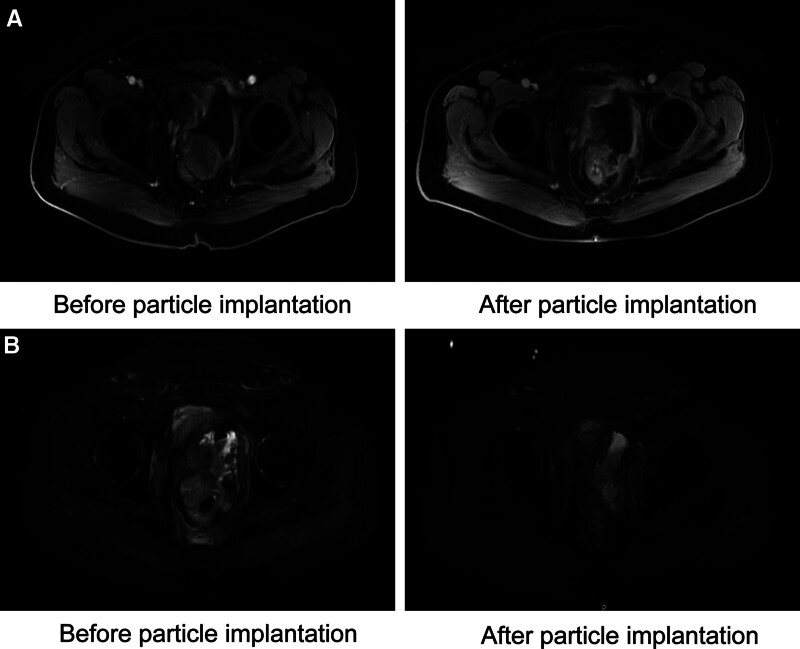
(A) MRI comparison of local recurrent pelvic lesion before I-125 seed implantation and after I-125 seed implantation for 2 months. (B) MRI comparison of second local recurrent pelvic lesion before I-125 seed implantation and after I-125 seed implantation for 3 months. I-125 = iodine-125, MRI = magnetic resonance imaging.
